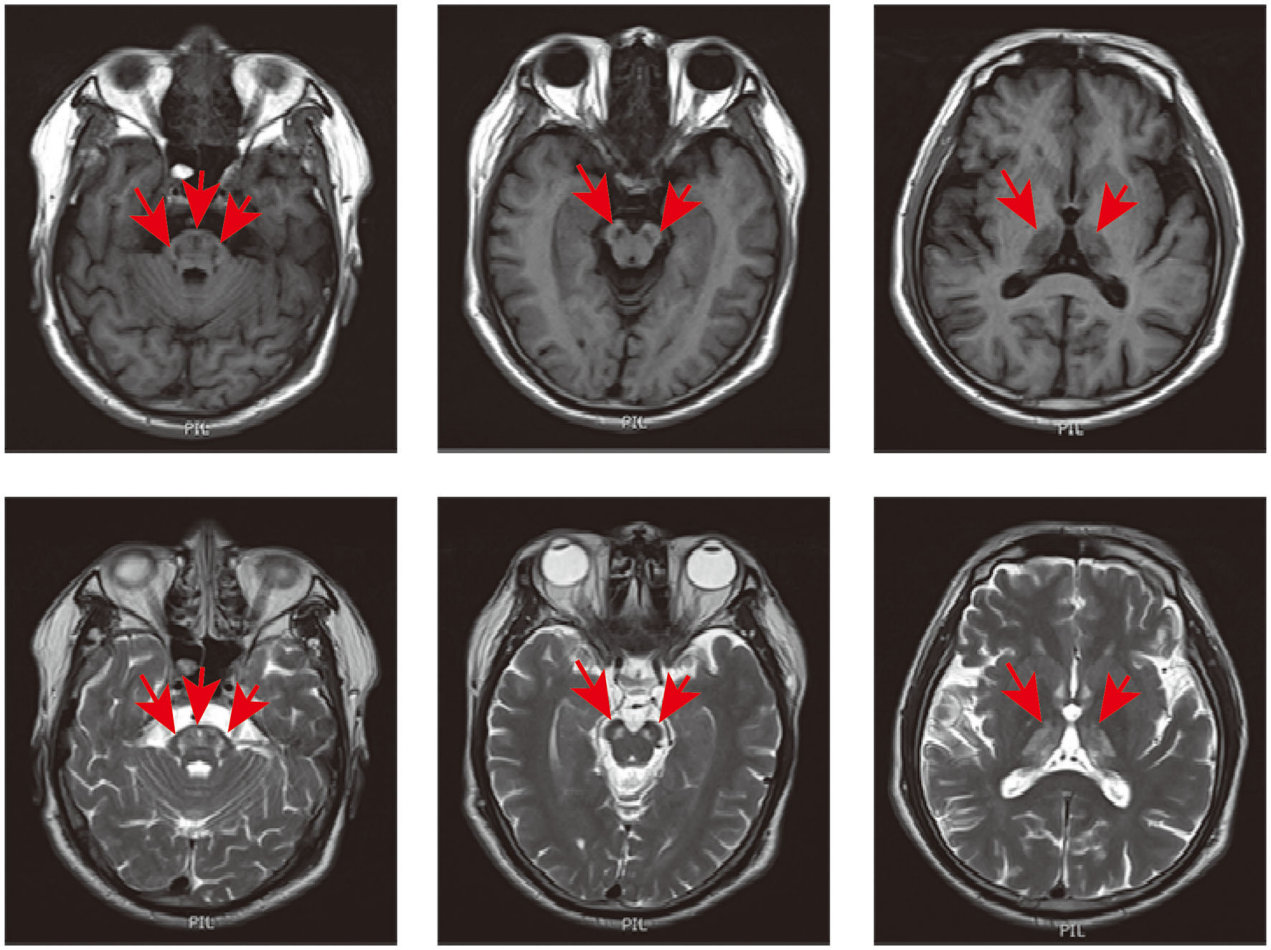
Cranial MRI of the patient. Axial T1 and T2-weighted imaging showed hypointense and hyperintense signals in the thalamus, mesocephalon, and pons (indicated in red arrow).
